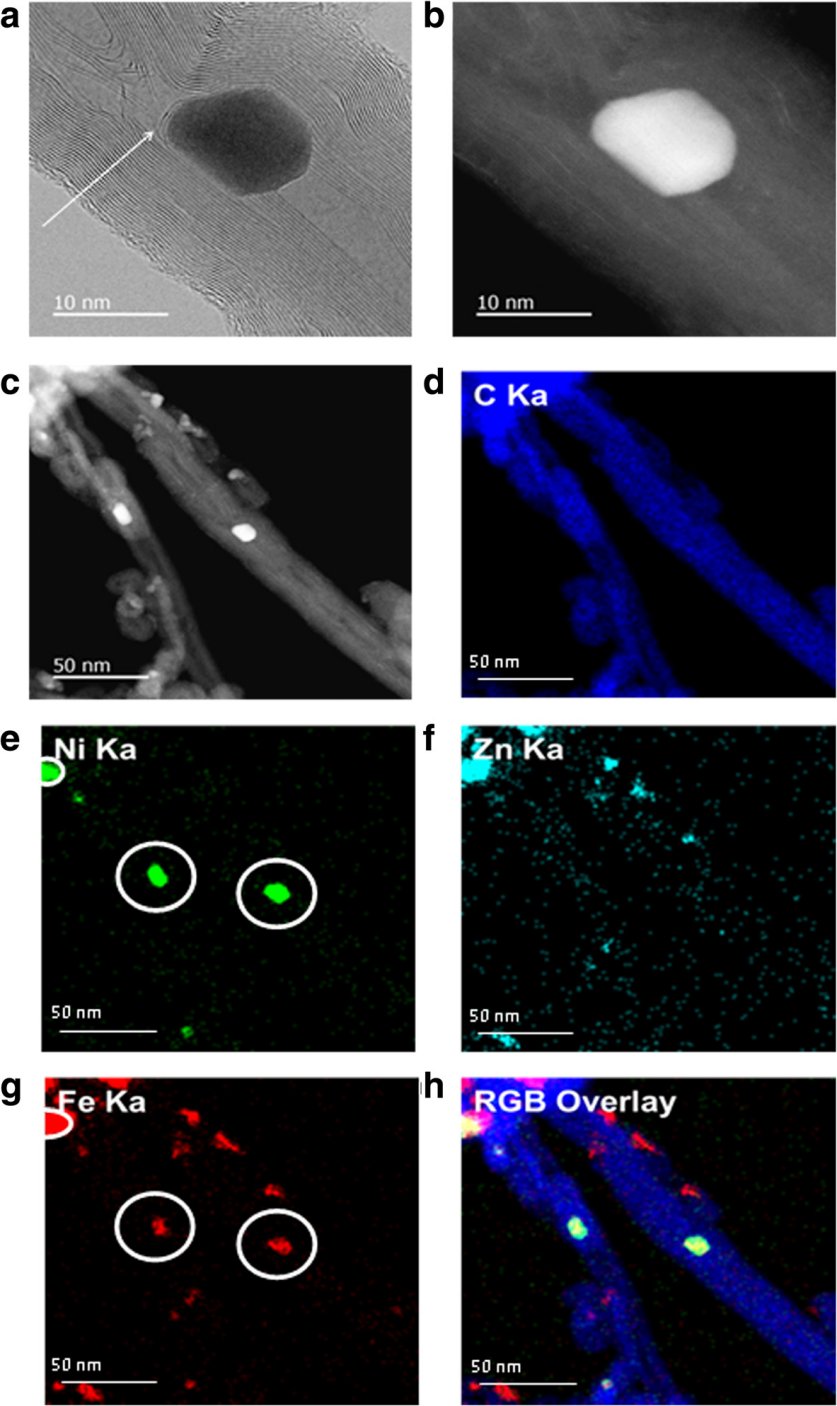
a STEM bright field image of single ZnFe_2_O_4_/mCNT. *Arrow* indicates the internal walls of mCNT partly coating ZnFe_2_O_4_ particle. b STEM dark field image of single ZnFe_2_O_4_/mCNT. c STEM annular dark-field image of ZnFe_2_O_4_/mCNTs. d The EDS x-ray maps of e nickel, the *circles* indicate Ni is agglomerated in the presence of ZnFe_2_O_4_. f Zinc. g Iron, the *circles* indicate the presence of iron in the same locations of Ni. h Display of the three specified maps as *red*, *green*, and *blue* overlays—representing iron, nickel, and carbon phase distributions
